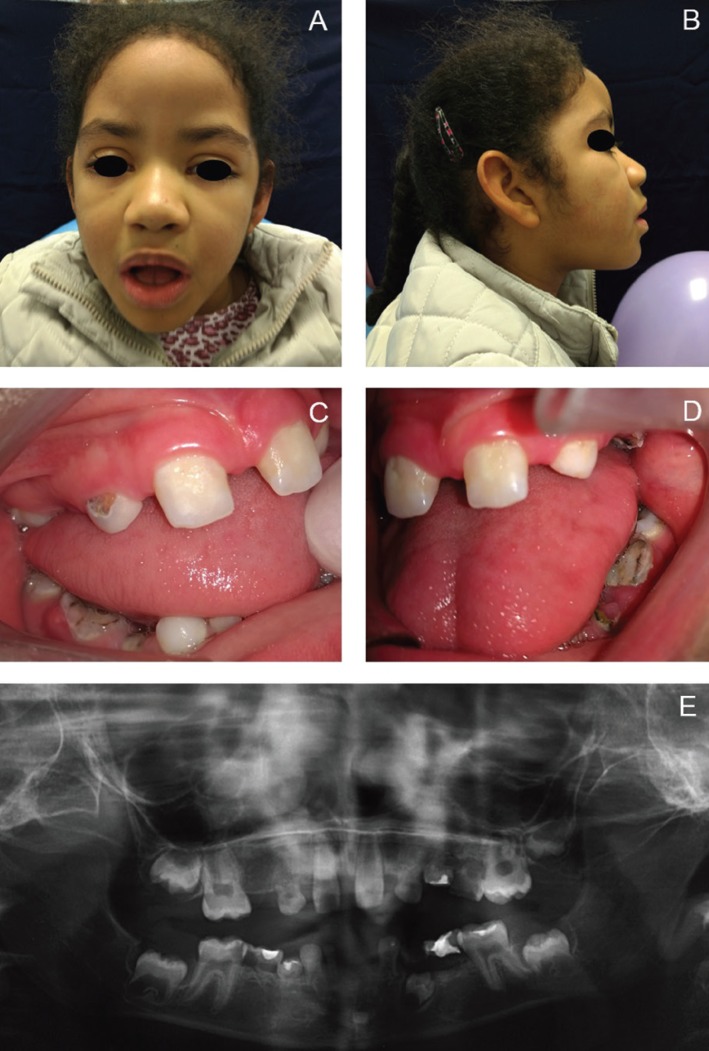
Patient #8. A) Frontal view of the hypotonic face showing long palpebral fissure with eversion of the lateral third of the lower eyelids, hypertelorism, palpebral ptosis, arched and sparse eyebrows in the lateral third, and wide nose. B) Lateral view of the face showing protruding ears with low implantation and depressed nasal bridge. C) Intra-oral right view showing spaced teeth, absence of teeth, enamel hypoplasia and caries lesions. D) Intra-oral left view showing spaced teeth, absence of teeth, enamel hypoplasia and caries lesions. D) Panoramic radiograph showing dental agenesis and prolonged retention of tooth #41.
